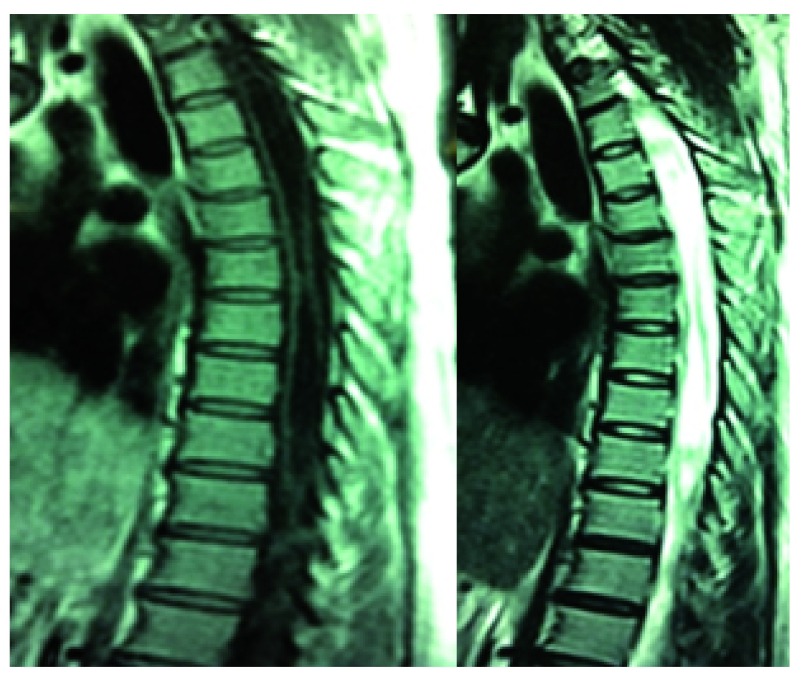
T1 and T2 MRI shows extension of syrinx up to lower T8 level.
